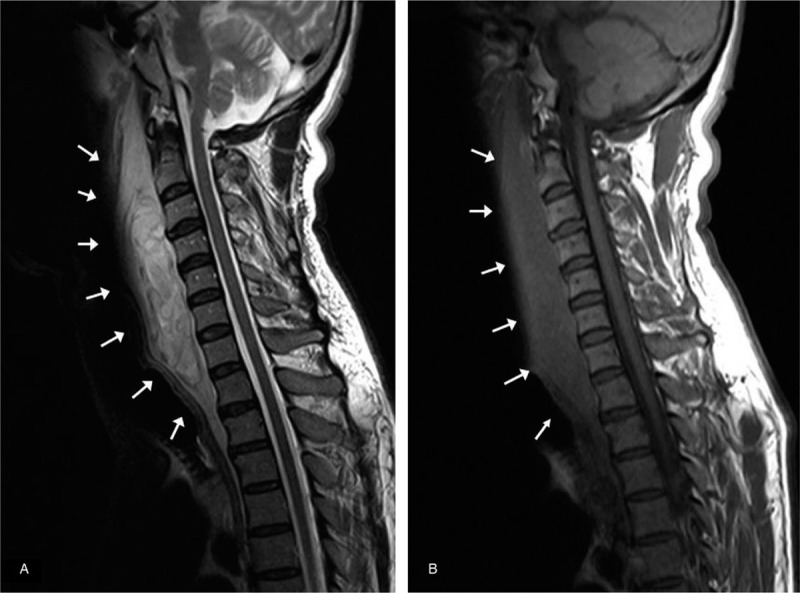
(a) T2-weighted sagittal Magnetic Resonance Imaging shows a longitudinal mass with heterogenous signal intensity in the retropharyngeal space (arrows). (b) T1-weighted sagittal Magnetic Resonance Imaging shows a mass with low signal intensity in the retropharyngeal space (arrows).
